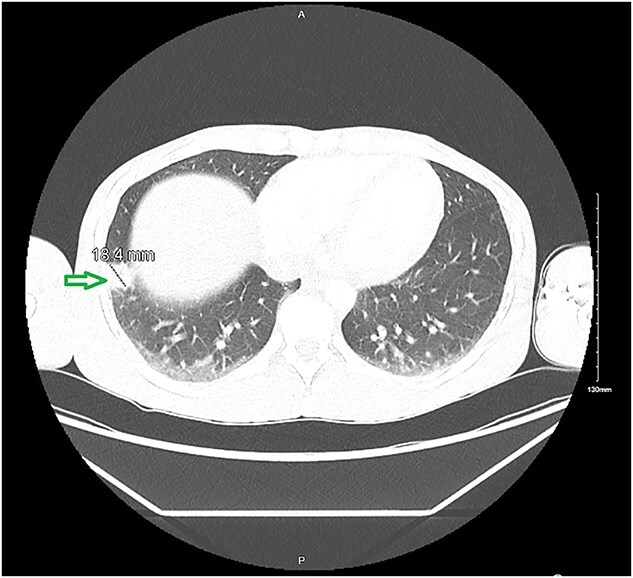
Axial CT scan of the chest demonstrating bilateral lung parenchyma. A focal peripheral lesion (green arrow) is seen in the left lung measuring approximately 18.4 mm, located subpleurally. Surrounding lung fields show patchy opacities, suggestive of associated inflammatory or infectious changes.
